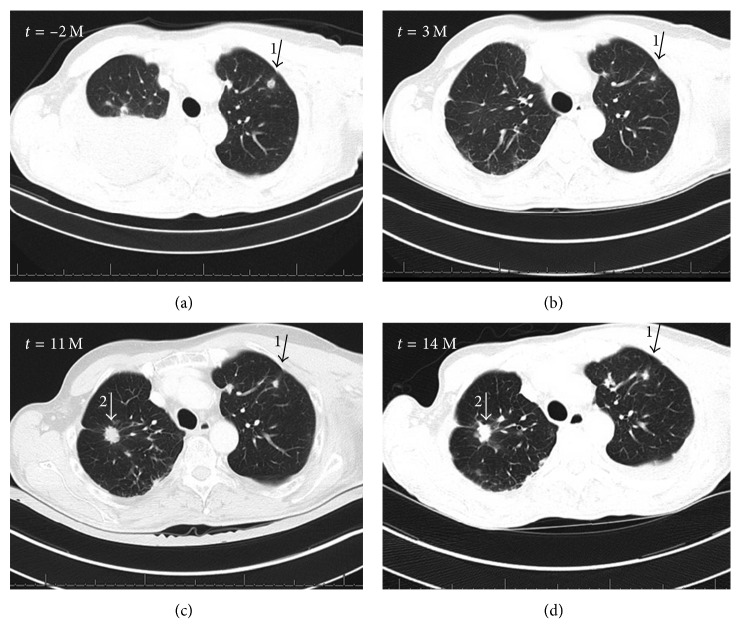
Computer tomography demonstrating pulmonary metastases two months before treatment (*t* = −2 M), with partial regression after 3 months of vismodegib treatment (*t* = 3 M, arrow 1) and with progressive disease after 11 and 14 months (arrow 1). Arrow 2 indicates new metastasis first visible on the CT scan after 11 months of treatment.
